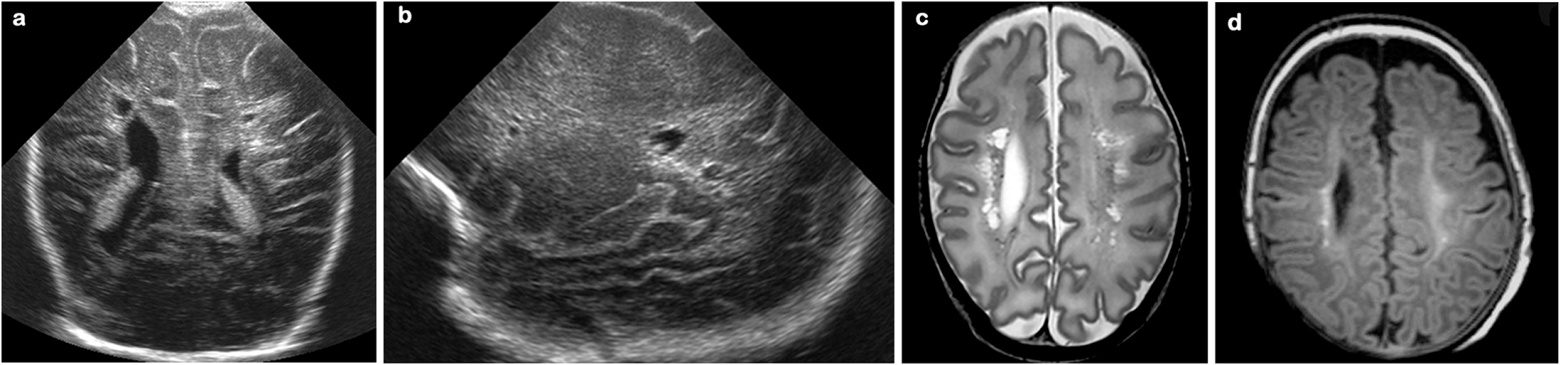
31 week gestation infant; cUS performed 4 weeks after birth. Coronal (a) and right parasagittal view (b) showing extensive cysts, more so on the right. (c) First T2-weighted MRI performed 4 weeks after birth showing extensive cysts, more prominent on the right. (d) The cysts were no longer seen on the T1-weighted TEA-MRI; extensive high SI changes and white matter loss are seen instead, also more marked on the right side.
